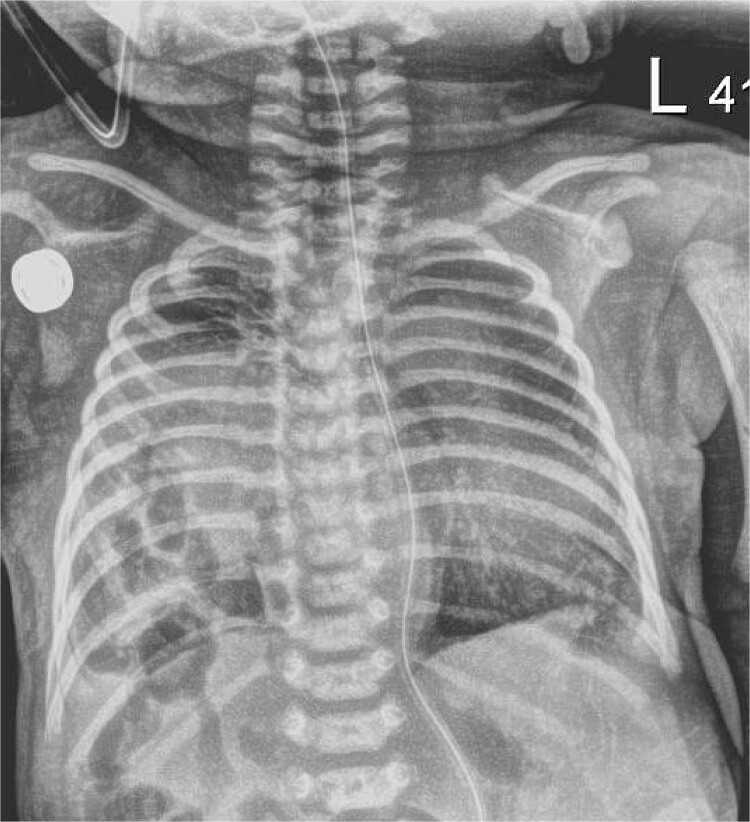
Chest X-ray, hour of life 1: there is a lucency in the right chest from the congenital diaphragmatic hernia as well as loops of bowel. There is no evidence of mediastinal shift and the superior aspect of the lung appears to aerate.
